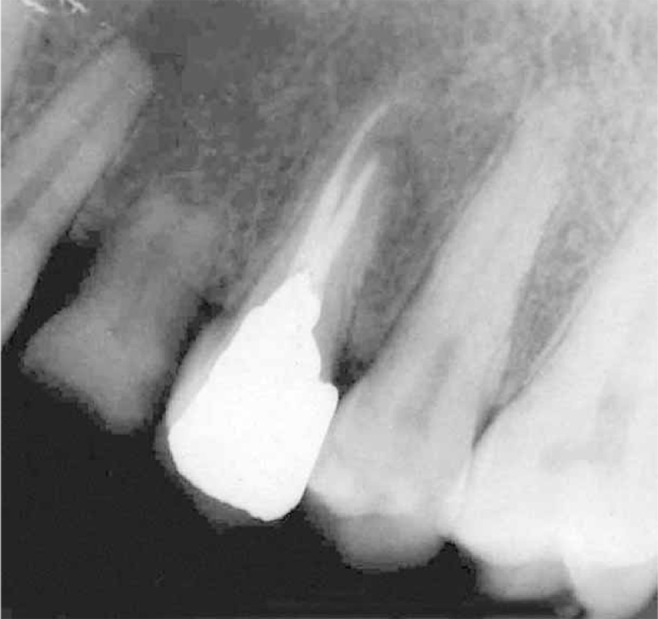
Periapical radiograph showing normal hard tissue after 2 years
